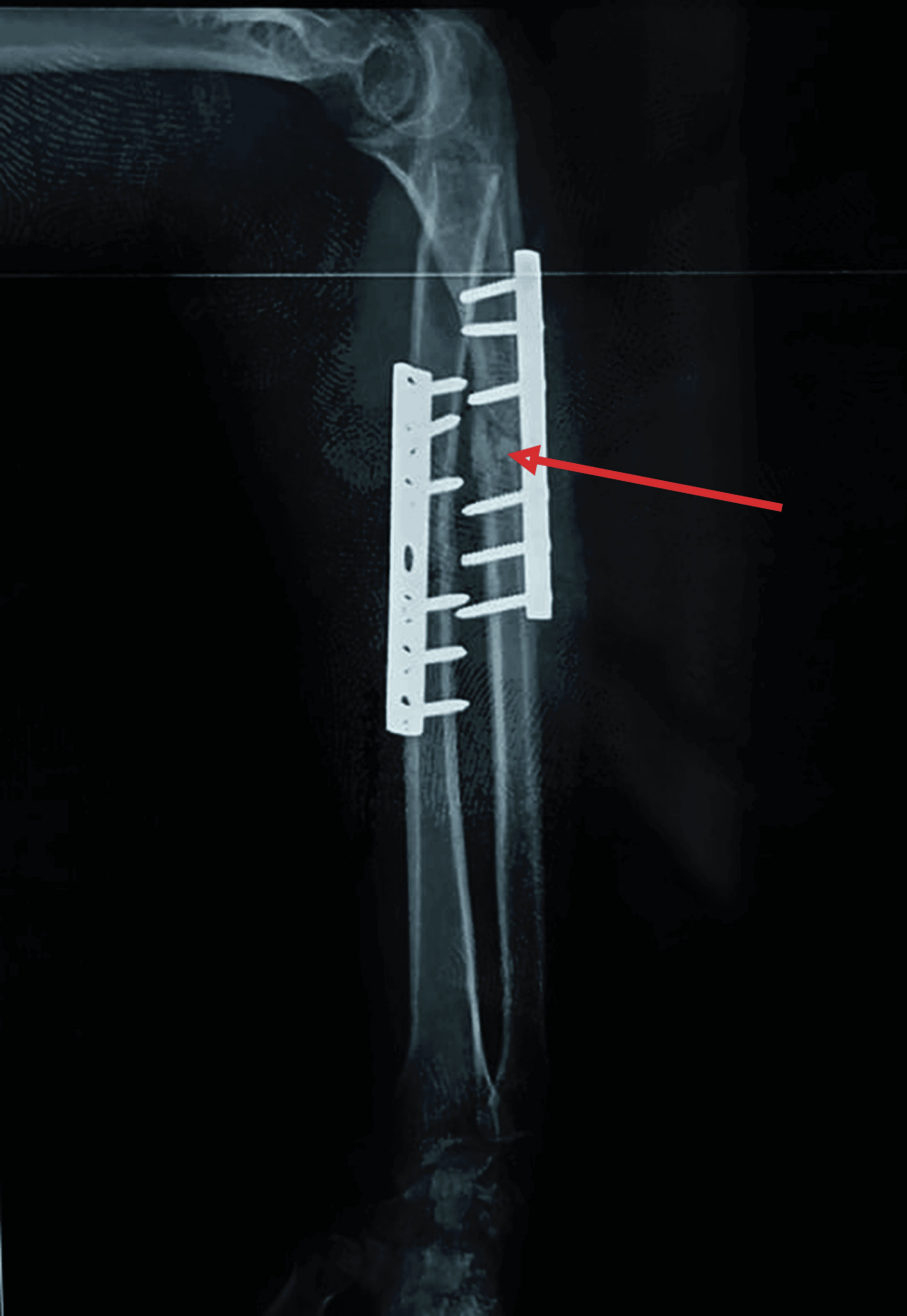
Left forearm radiograph showing evidence of fracture union
